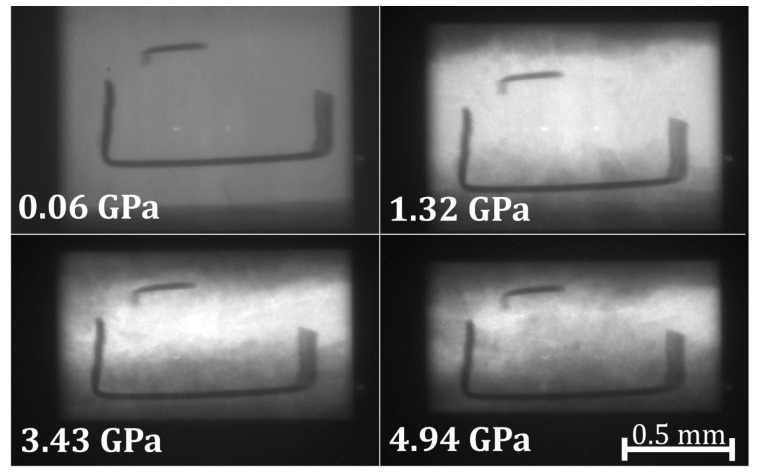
X-ray radiography images of the reprocessed borosilicate glass sample to 4.94 GPa.
